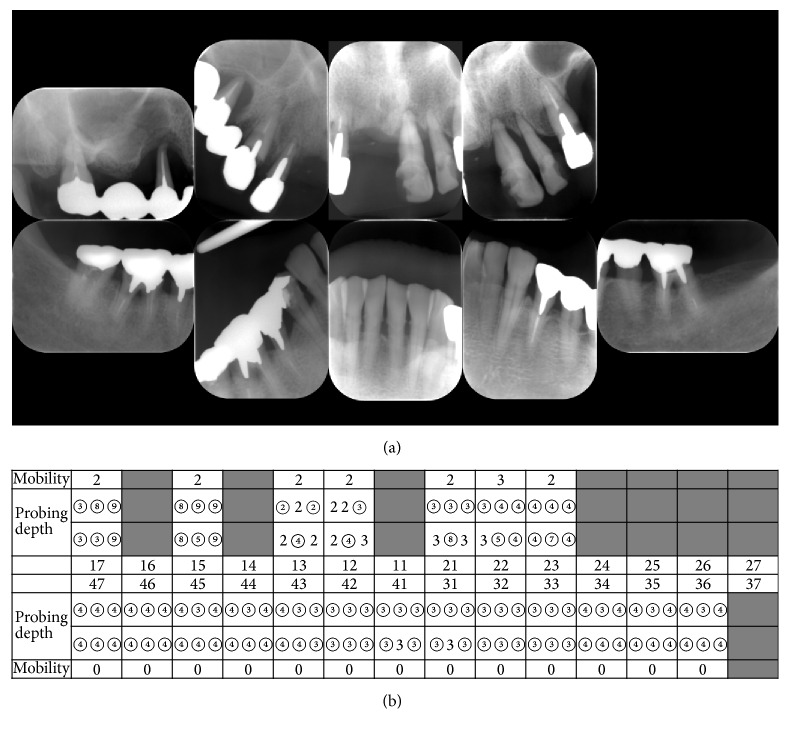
(a) X-ray images and (b) periodontal chart at initial dental visit. Circle on number indicates bleeding on probing.
